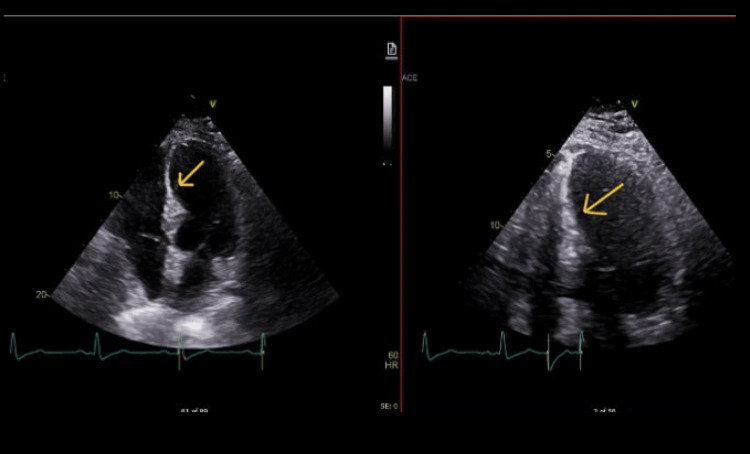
Echocardiogram from March 2022. Yellow arrows pointing to the interventricular septum. Transthoracic echocardiogram (TTE) complete with contrast, bubble, strain, and 3D PRN order panel (March 2022). Acquisition window and orientation are an apical five-chamber view, utilizing the Change Healthcare Cardiology Software (CPACS) as the imaging tool/imaging mode. Left ventricle (LV) cavity size is normal. The visually estimated ejection fraction is 70%. There are no regional LV wall motion abnormalities noted. LV mass and mass index (2D) are 98 g and 42 g/m^2^, respectively. The LV wall thickness is noted as normal. The LV outflow tract (LVOT) stroke volume and LVOT stroke index are 136 g and 59 mL/m^2^, respectively. No significant changes were observed compared to previous imaging. Imaging obtained from centralized electronic medical records with the consent of the patient.
